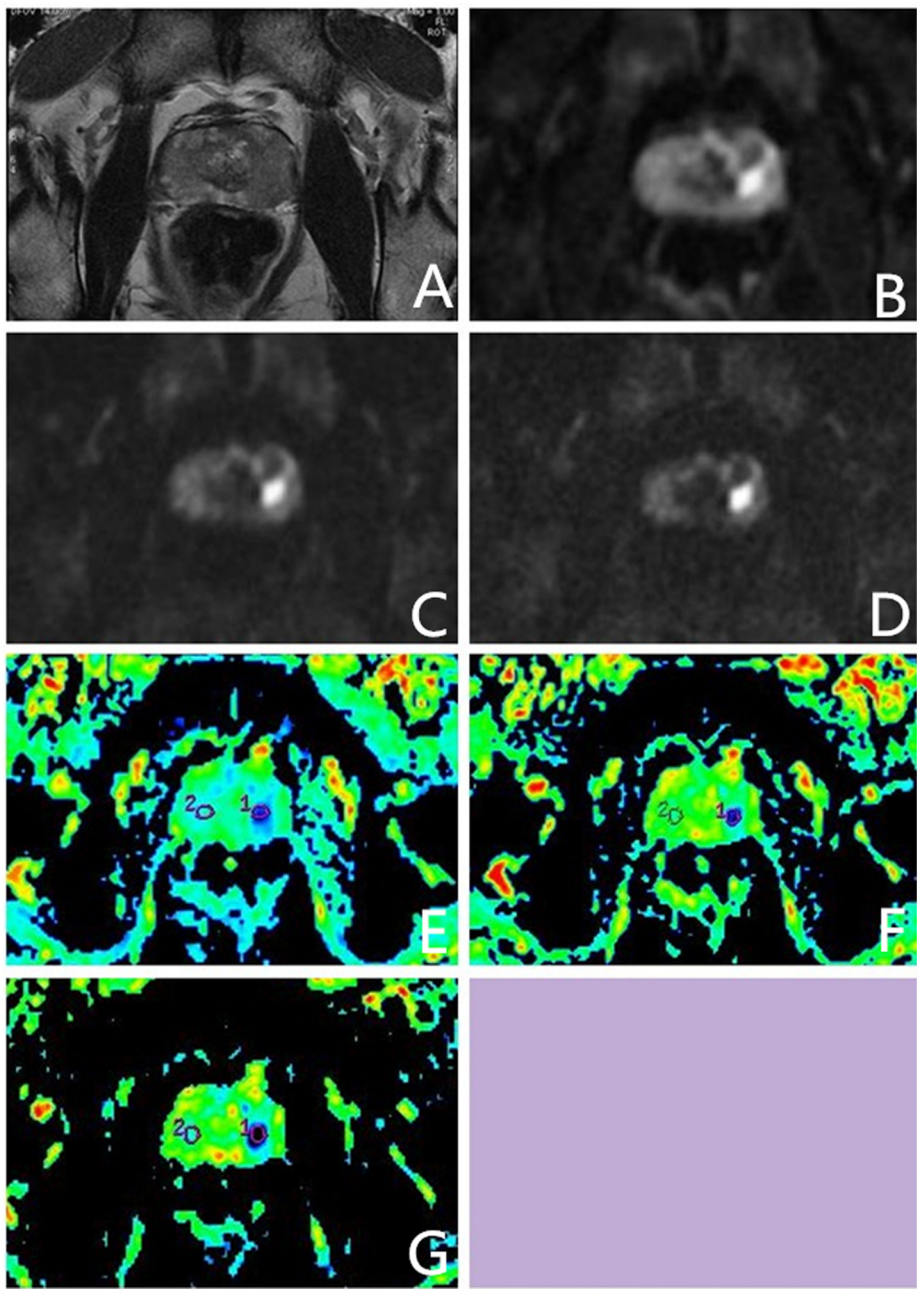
Images from a 55 yr old male patient with total prostate-specific antigen (PSA) 3.41 ng/ml and free PSA 0.247ng/ml. Systematic transrectal biopsy confirmed. prostate cancer (Gleason score 4 + 3 = 7). (A) High-solution T2-weighted imaging shows low signal intensity area in the left boundary region of the transition zone (TZ) and peripheral zone (PZ); (B–D) On ultrahigh b-value diffusion-weighted imaging (DWI) (b = 1000, 2000, 3000 s/mm^2^), lesions on the left showed significantly higher signal nodules. Increase in b-values reduced normal tissue signal intensity, but not signals from prostate cancer tissue; (E–G) With conventional and ultrahigh b-value DWI (b = 1000, 2000, 3000 s/mm^2^), apparent diffusion coefficient map (ADC), and organization of lesions on the left and normal ADC values were significantly different; lesion ADC values decreased with increase in b-value. Key: ADC—apparent diffusion coefficient; DWI—diffusion-weighted imaging; PSA—prostate-specific antigen; PZ—peripheral zone; TZ—transition zone.
